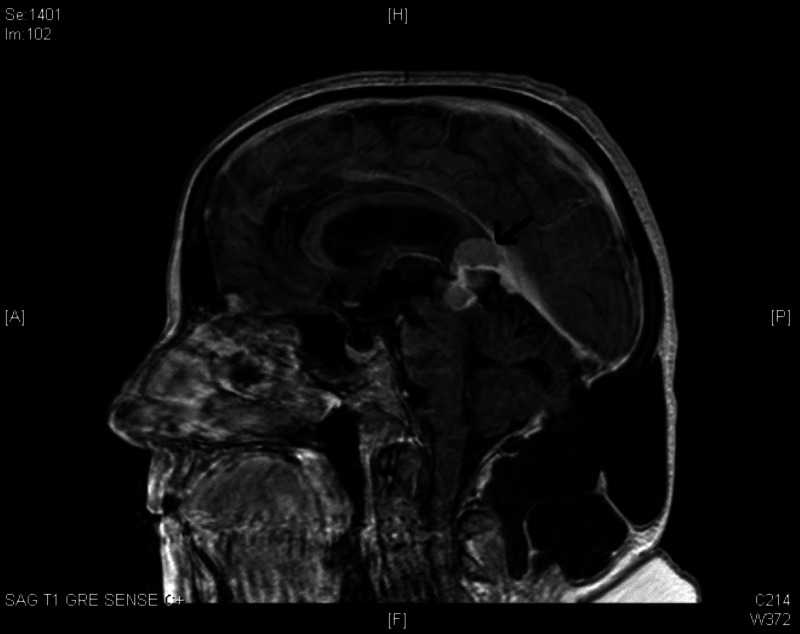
Postoperative MRI showing a small remnant tumor with pseudomeningocele MRI: magnetic resonance imaging
